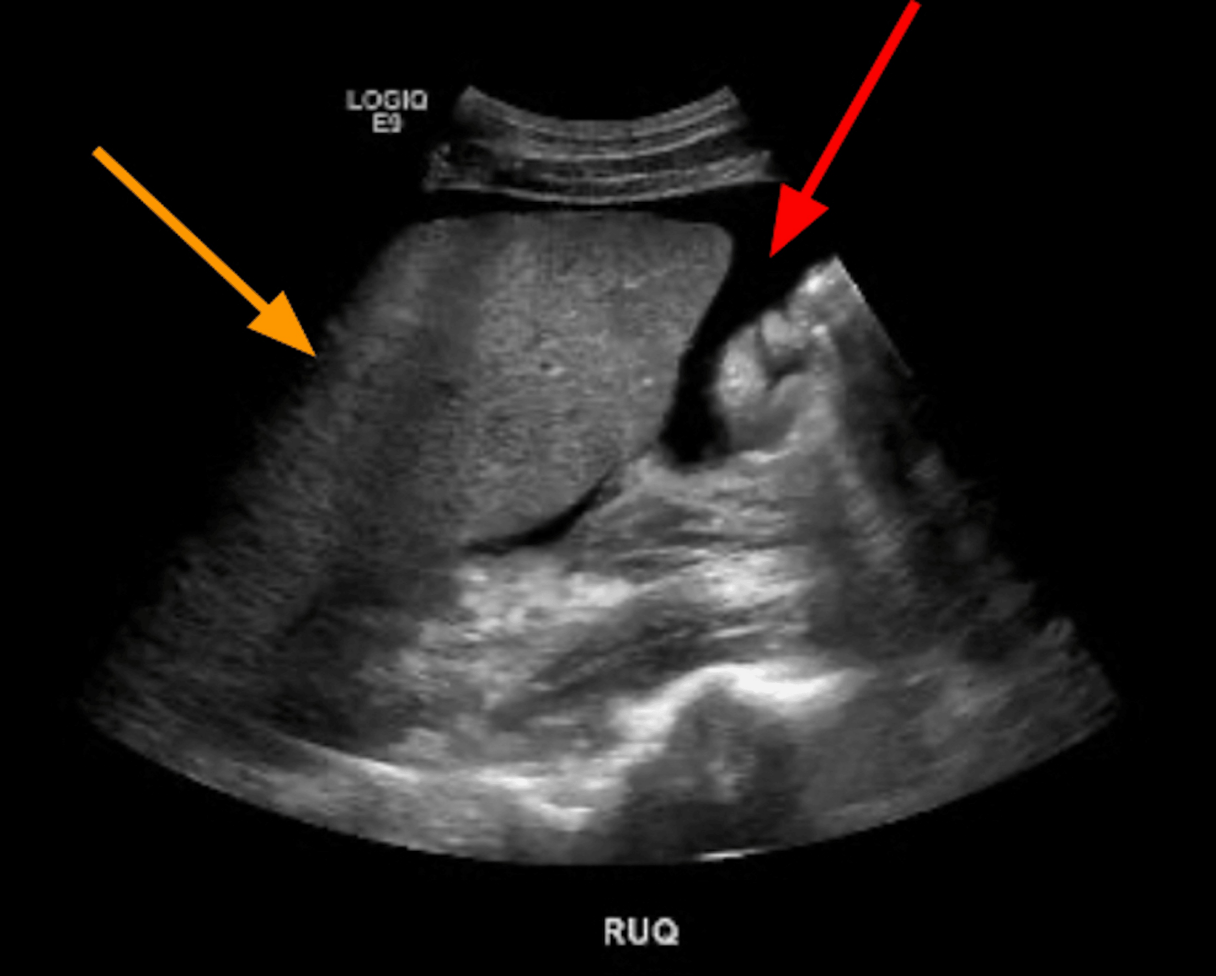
Complete abdominal ultrasound The image displays excessive abdominal ascites (red arrow), with hepatomegaly also observed (orange arrow)
